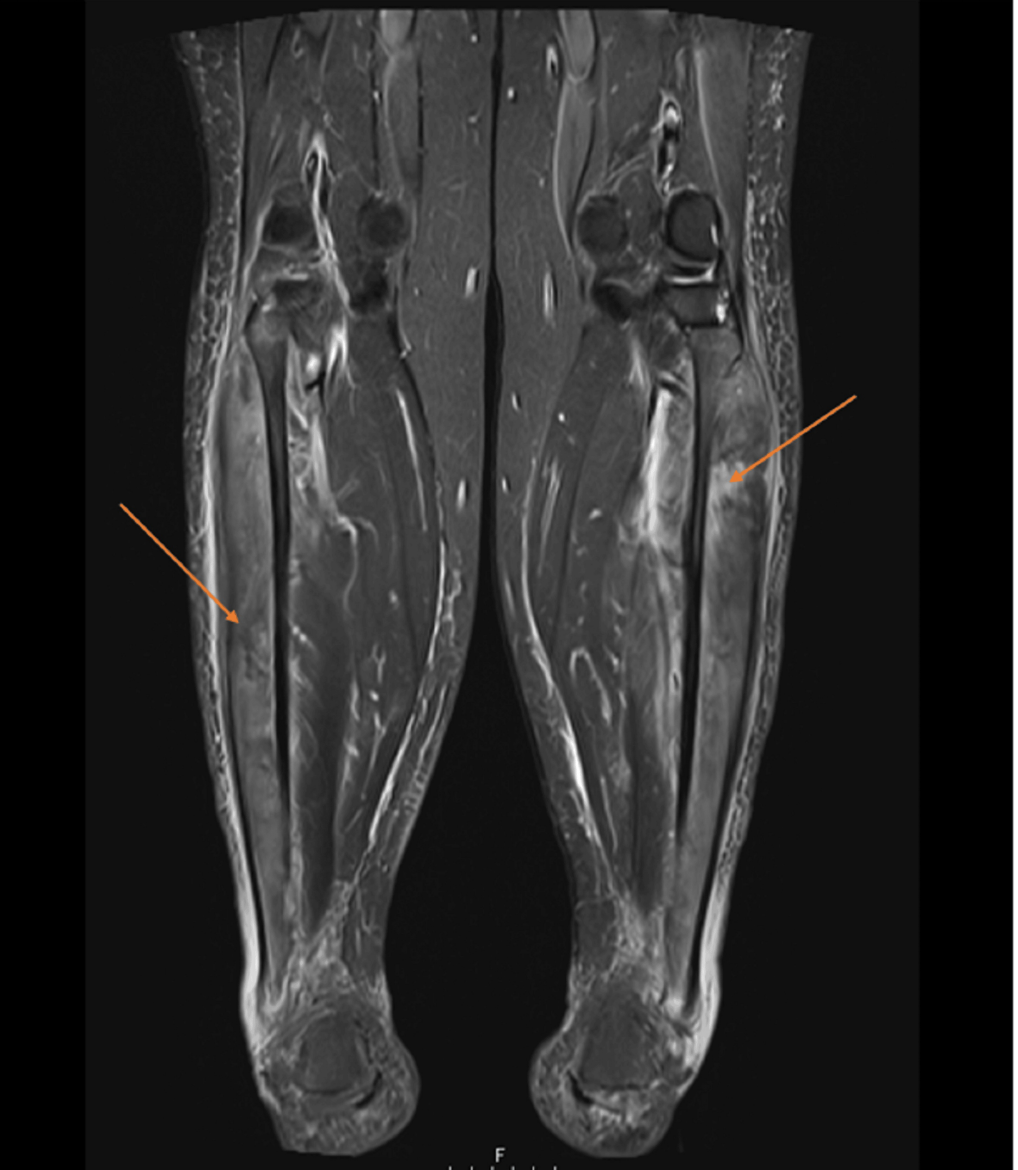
MRI calves coronal view. T2 hyperintensity consistent with muscle edema (arrows). MRI, magnetic resonance imaging
